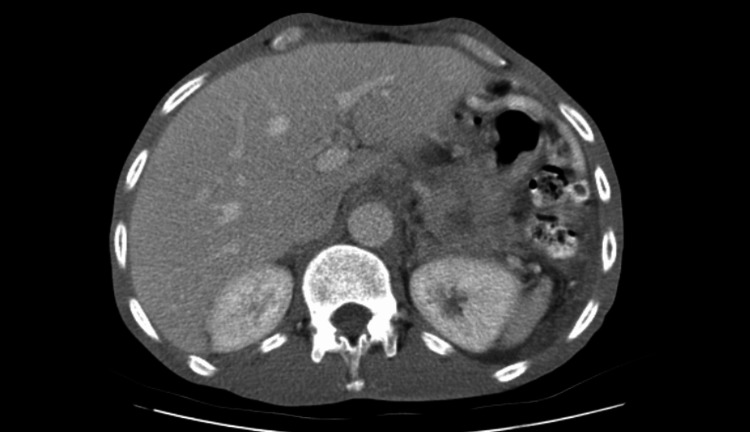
CT of the abdomen with oral and intravenous contrast. Heterogenous and hypoattenuating mass in the distal pancreas measuring 4 cm in diameter.
